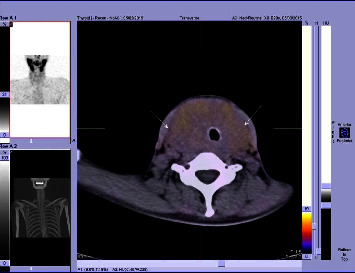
M99Tc SPECT CT scan showing the profusely enlarged goiter with retrotracheal disposition. The trachea is compressed and displaced to the right.
